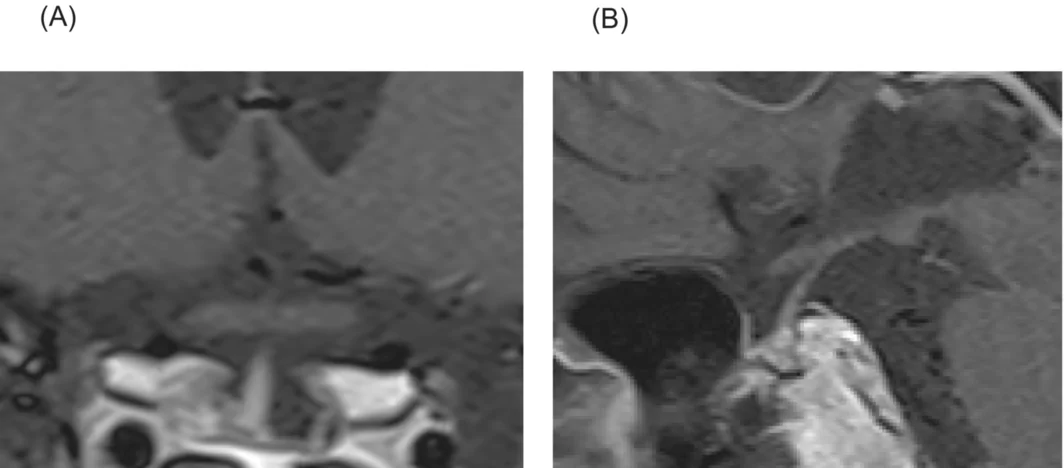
Pituitary magnetic resonance imaging (MRI). (A) Coronal section. (B) Sagittal section. Pituitary gadolinium‐enhanced T_1_‐weighted MRI demonstrating an empty sella. The pituitary stalk is deviated to the right. A deformation of the posterior clinoid process is identified.
